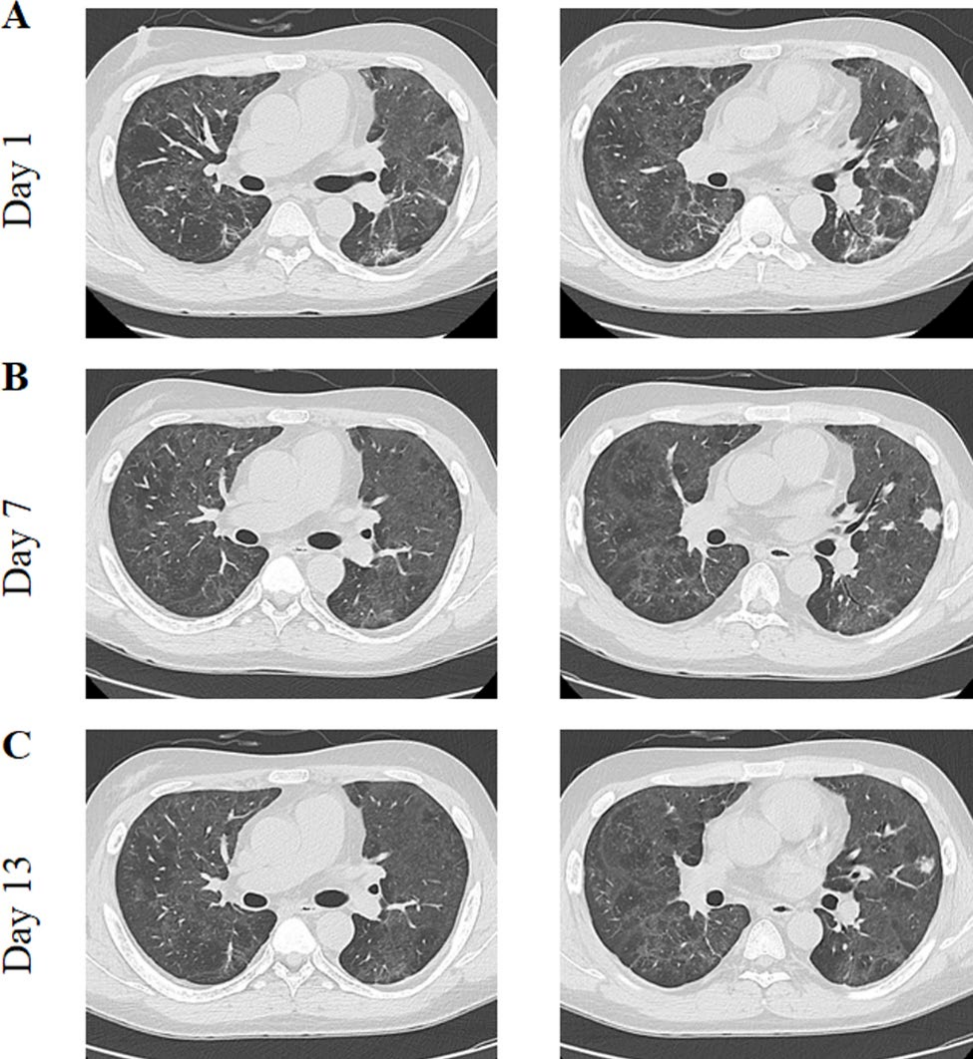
Case 3-Computed tomography images at different hospital days
